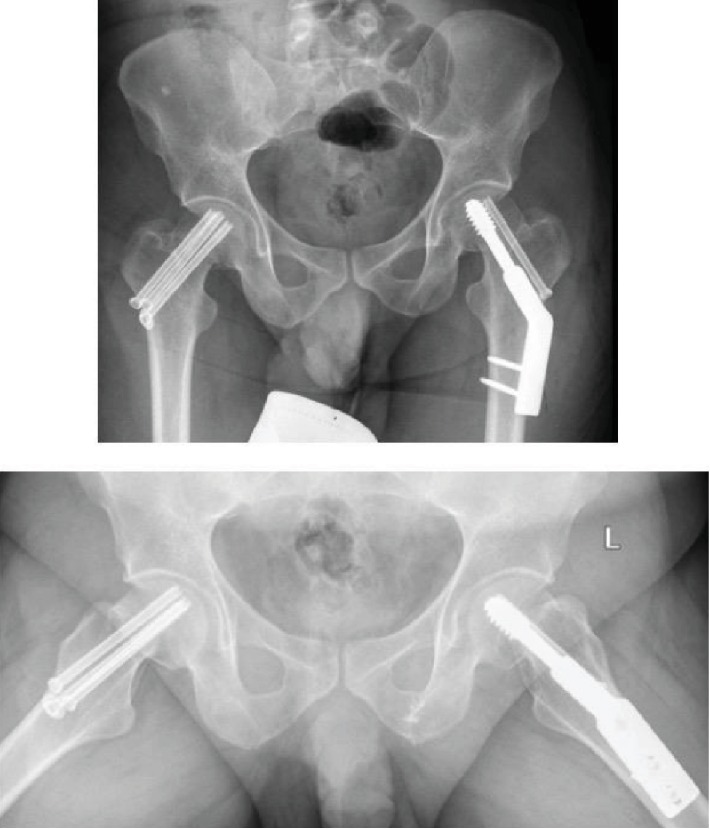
18-month follow-up images showing radiographic union of both sides with no signs of avascular necrosis.
